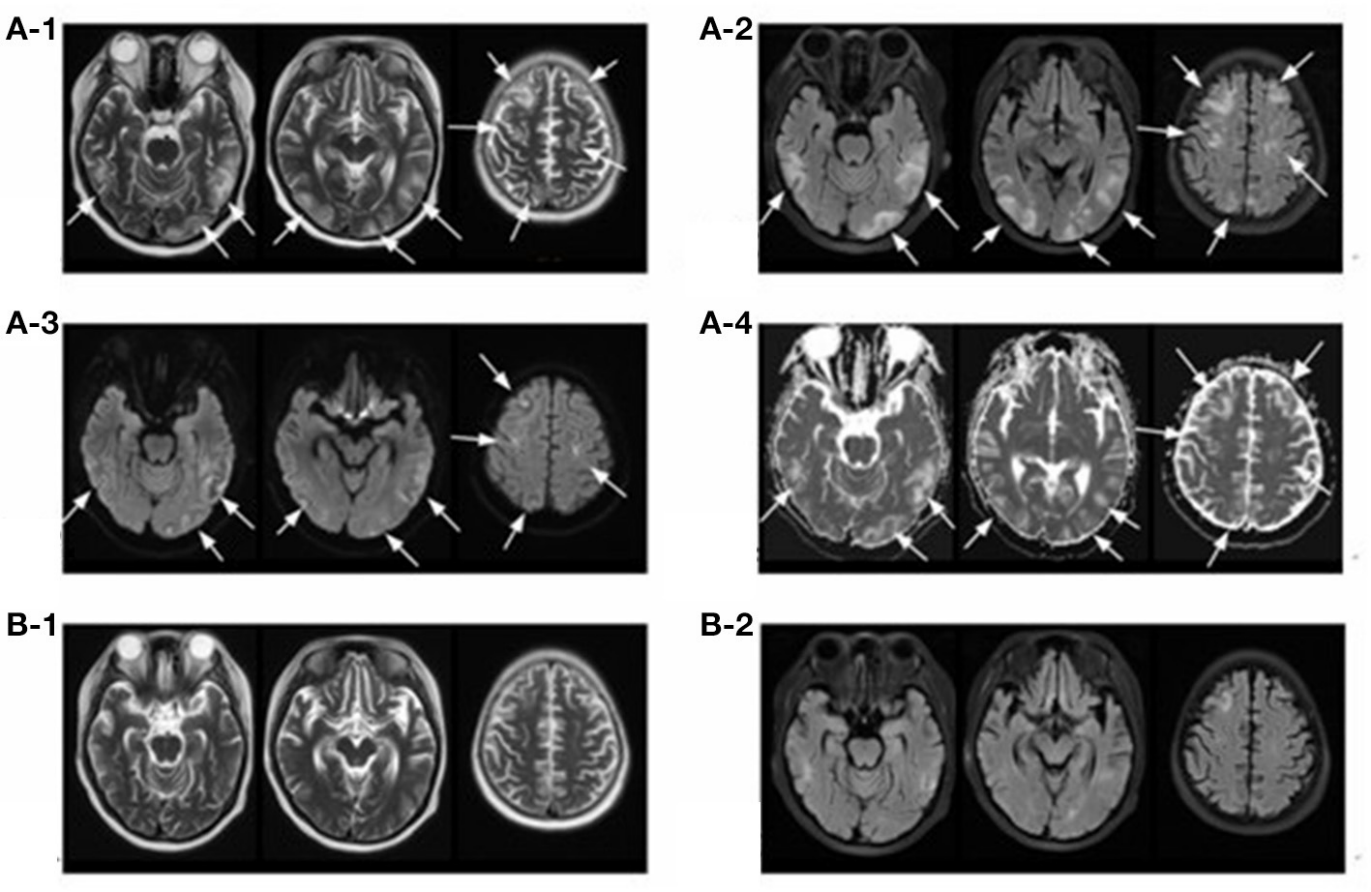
Magnetic resonance imaging (MRI) of brain. T2-weighted (A-1) and FLAIR (A-2) sequences depict hyper-intense lesions involving the bilateral cerebral hemispheres (occipital lobe, parietal lobe, temporal lobe and frontal lobe) and cerebral cortex and subcortex (arrows), most of which disappeared 17 days later (B-1,B-2). Diffusion-weighted magnetic resonance imaging (A-3) and apparent diffusion coefficient (A-4) sequences of the involved regions showed isointense or hyperintensity lesions (arrows).
